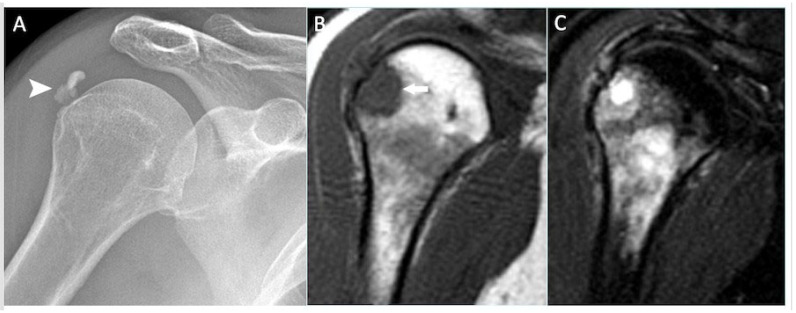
Shoulder anteroposterior radiograph (A), T1-weighted (B), and STIR coronal MRI (C) demonstrating calcific tendinitis of the supraspinatus tendon (arrowhead), with intraosseous migration (white arrow) and florid intramedullary oedema.
